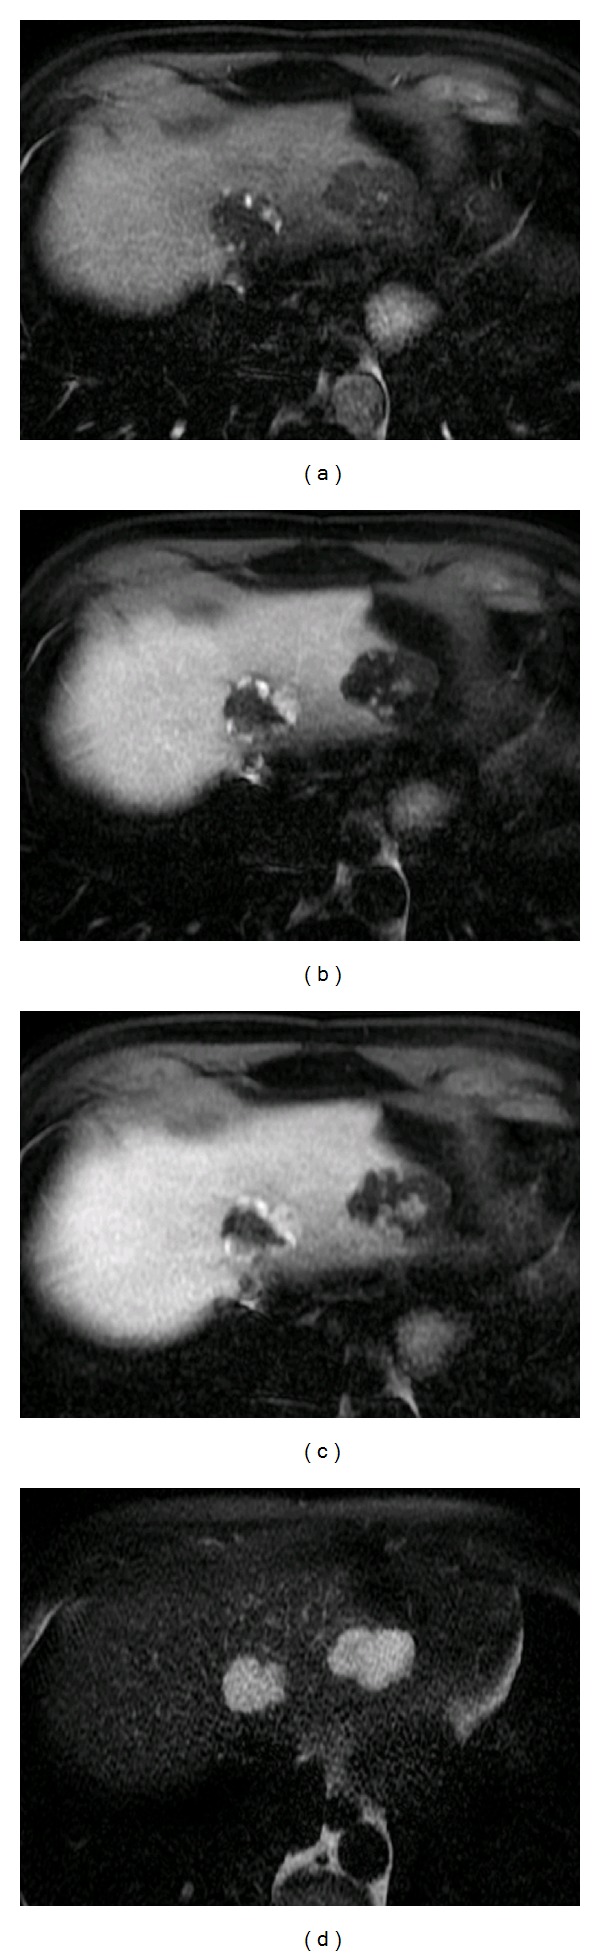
Two hemangiomas in gadolinium enhanced MRI (contrast medium: gadoxetic acid; disodium salt (Primovist, Eurokontrast GmbH, Heidelberg), scanner: GE Signa HDxt 1,5T (General Electric Company, USA)): peripheral nodular enhancement in T1 FS early arterial contrast phase (upper left): (T1 LAVA FS dynamic FA80, TR 185 TE 4,2), progressive centripetal enhancement in T1 FS late arterial (upper right): (T1 LAVA FS dynamic FA80, TR 185 TE 4,2) and portal-venous phase (lower left): (T1 LAVA FS dynamic FA80, TR 185 TE 4,2). Typical ill-shaped intermediate (less than in cysts) hyperintensity in T2 (lower right): (T2 FRFSE FS FA 90 TR 2500 TE 94,16). Lesion in left lobe is partially clotted with thrombosis and shows less enhancement.
